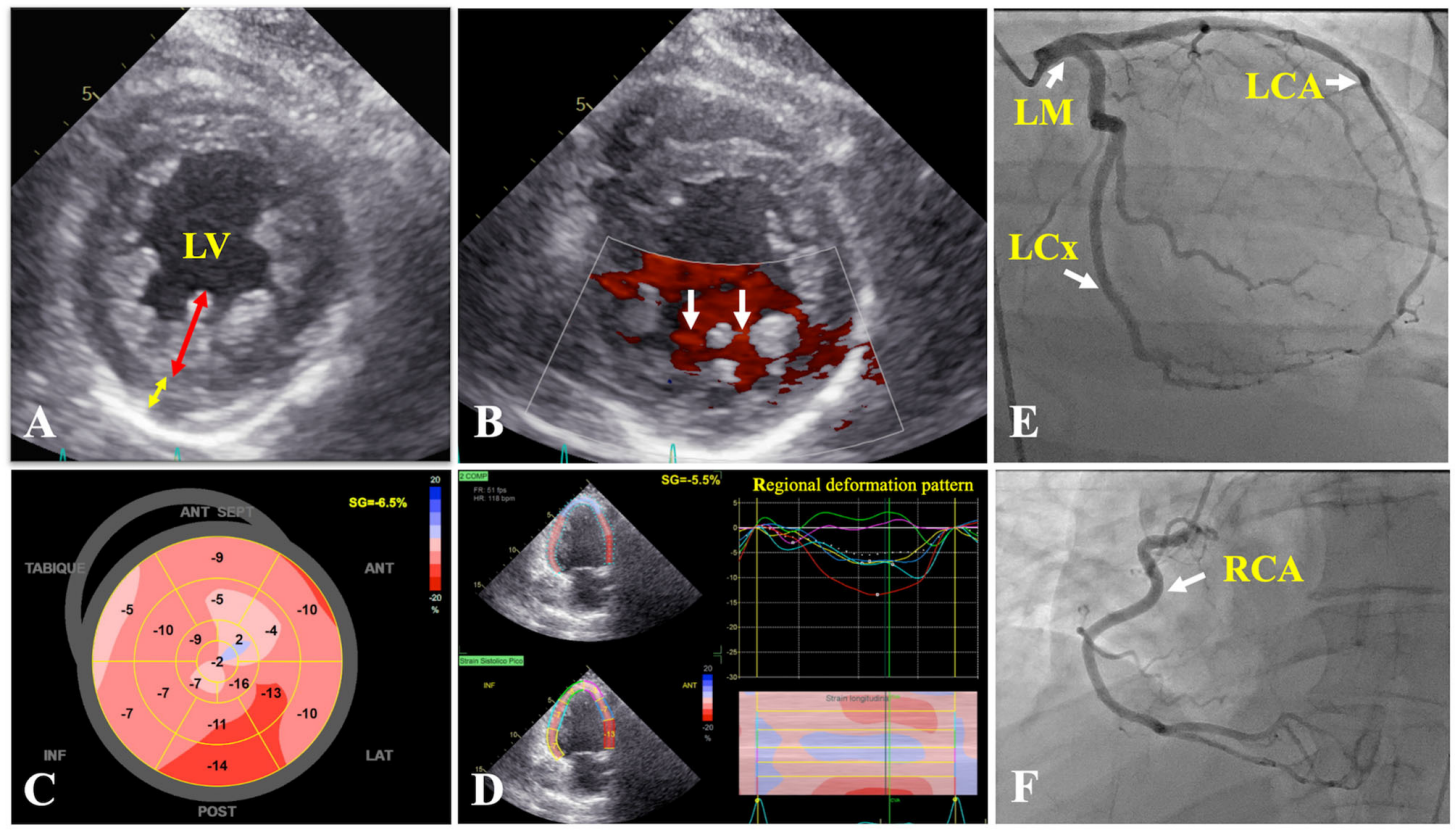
Transthoracic echocardiogram. (A) Parasternal short axis of the mid left ventricle demonstrating two-layer myocardial structure, with a ratio of ticker non-compacted (redline) to thin compacted (yellow line) myocardium >2 at end-systole in the inferolateral segment. (B) Flow between intertrabecular recesses by intraventricular blood on color Doppler (white arrows). (C) Reduced global longitudinal strain (−6.5%). (D) Two-chamber view showing a regional deformation pattern with a markedly decreased myocardial deformation in apical segments (green and purple curves) in comparison to basal segments (red and yellow curves). Coronary angiography: coronary arteries without lesions (E,F) (white arrows). LCA, left coronary artery; LCx, left circumflex coronary artery; LM, left main coronary artery; LV, left ventricle; RCA, right coronary artery.
